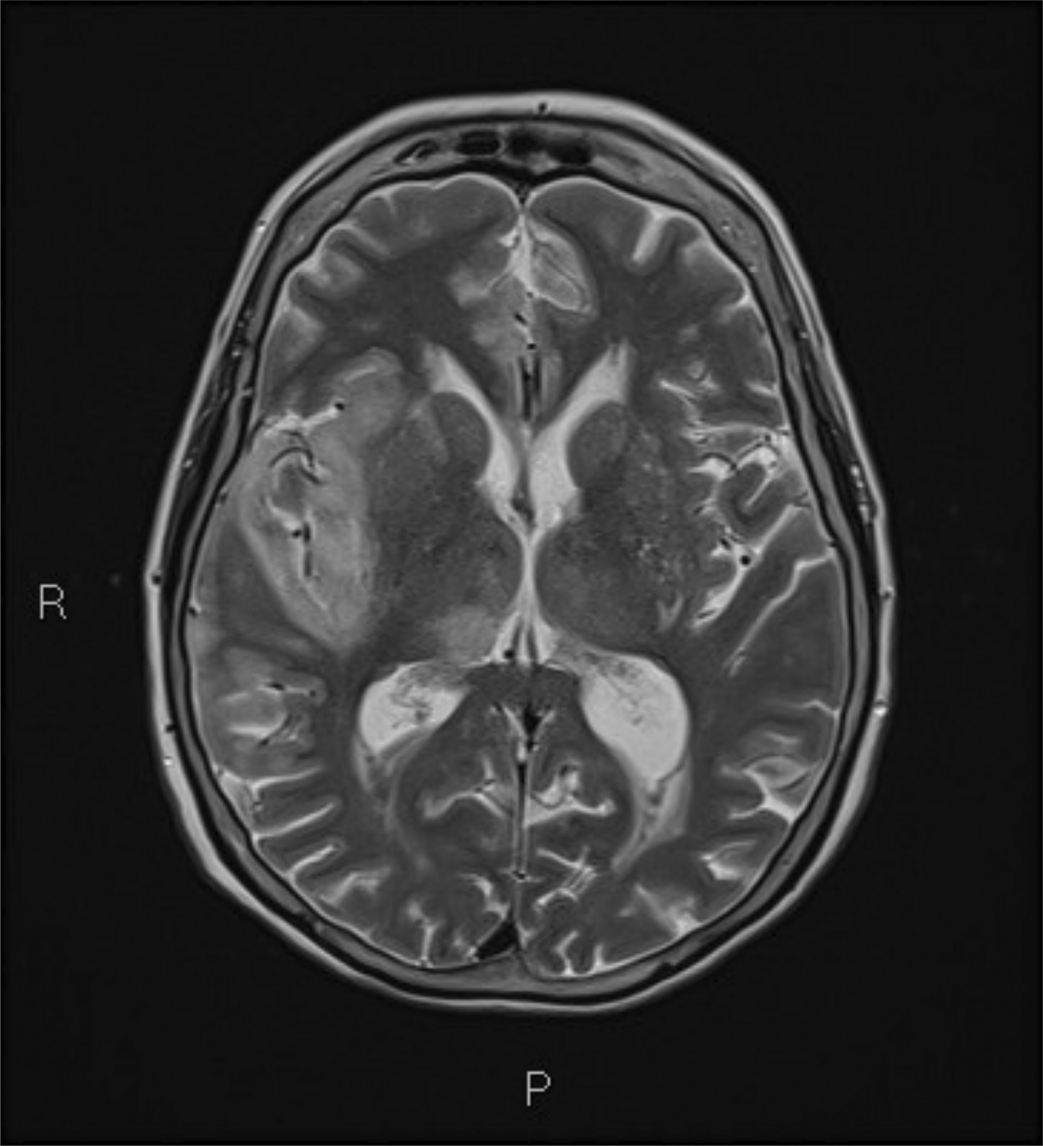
Magnetic resonance imaging (MRI) demonstrating generalized oedamatous changes of the right temporal lobe with moderate intensity restricted diffusion seen involving the gyri of the right temporal lobe and right insular regions.
